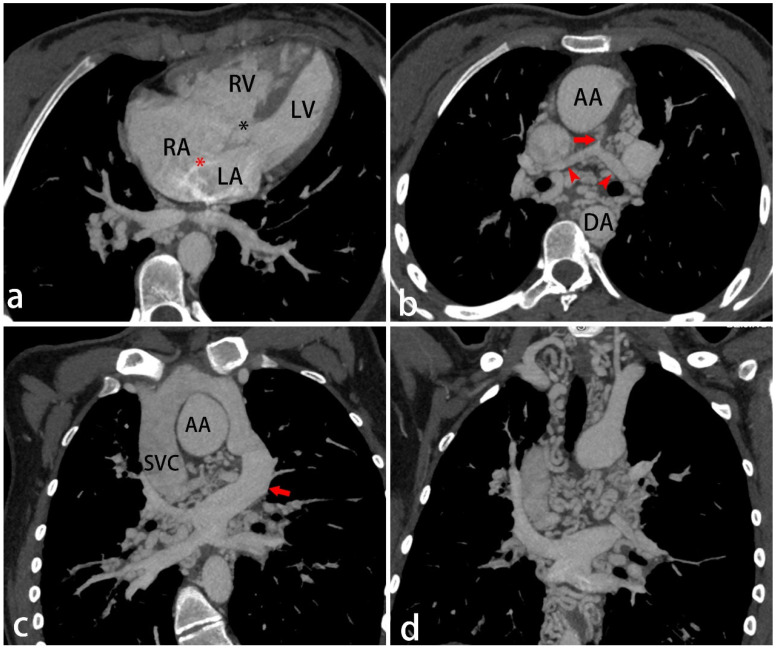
A 16-year-old female with pulmonary atresia with ventricular septal defect. Axial CT angiography image shows atresia of the main pulmonary artery ((b), red arrow) and hypoplastic left and right pulmonary arterial trunks ((b), red arrowheads), showing “seagull in flight” appearance. The four-chamber view shows a ventricular septal defect ((a), black asterisk) and an atrial septal defect ((a), red asterisk). There is anomalous pulmonary venous drainage into the superior vena cava ((c), red arrow). Multiple systemic collateral vessels are seen within the mediastinum (d). LV, left ventricle; LA, left atrium; RV, right ventricle; RA, right atrium; AA, ascending aorta; DA, descending aorta; SVC, superior vena cava.
